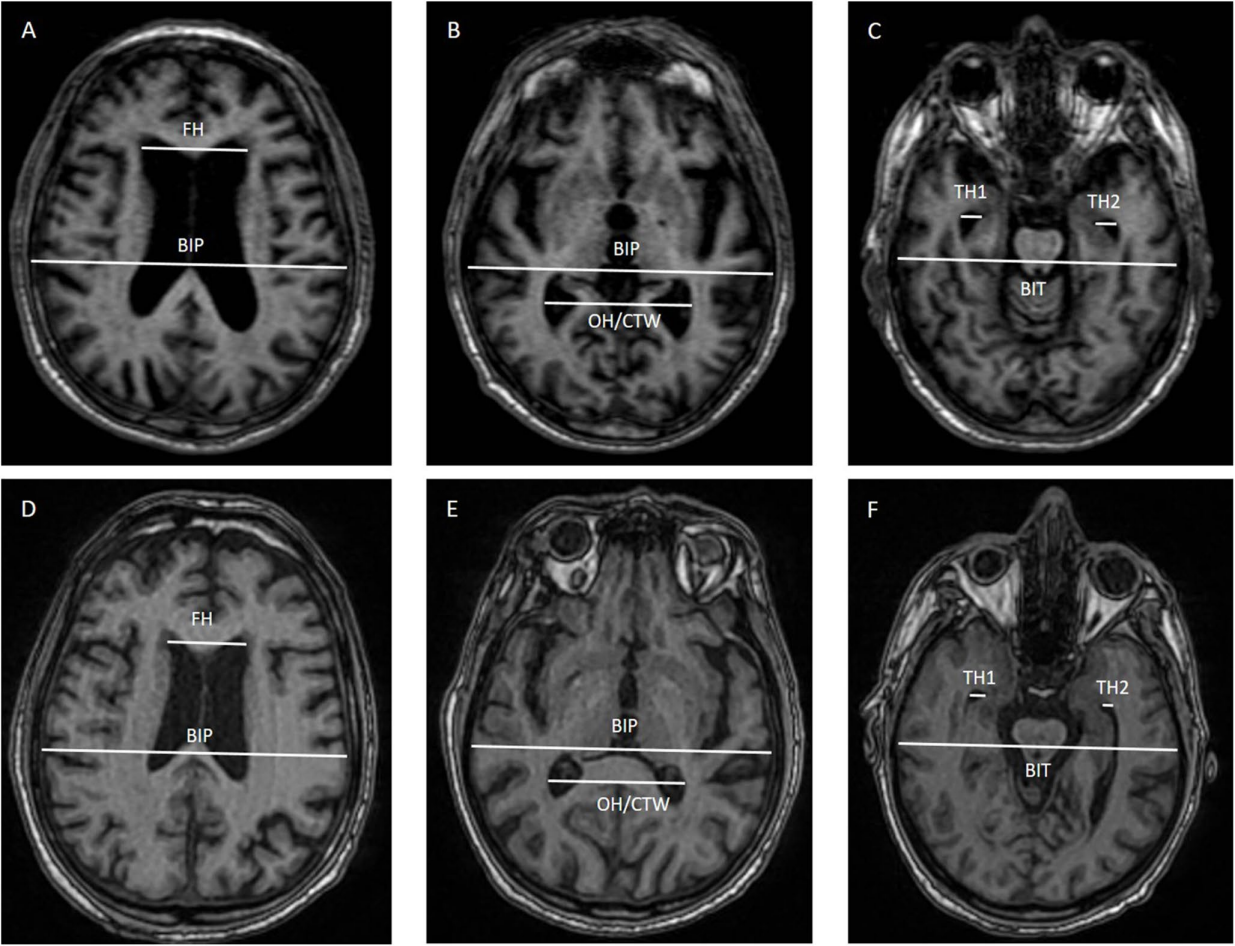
Linear measurement calculated on axial T1-weighted MRI computation of (A, D) Evan’s Index (EI), (B, E) Magnetic Resonance Hydrocephalic Index (MRHI) or Parieto-occipital Ratio (POR) and (C, F) temporal ratio (TR) to obtain Brain Linear Measurement (BLM) index for iNPH (figures above) and PSP patients (figures below). Abbreviations: FH, frontal horns; BIP: bi-parietal inner table; OH: occipital horns; CTW: collateral trigones width; TR: temporal horn; BIT: bi-temporal inner table
